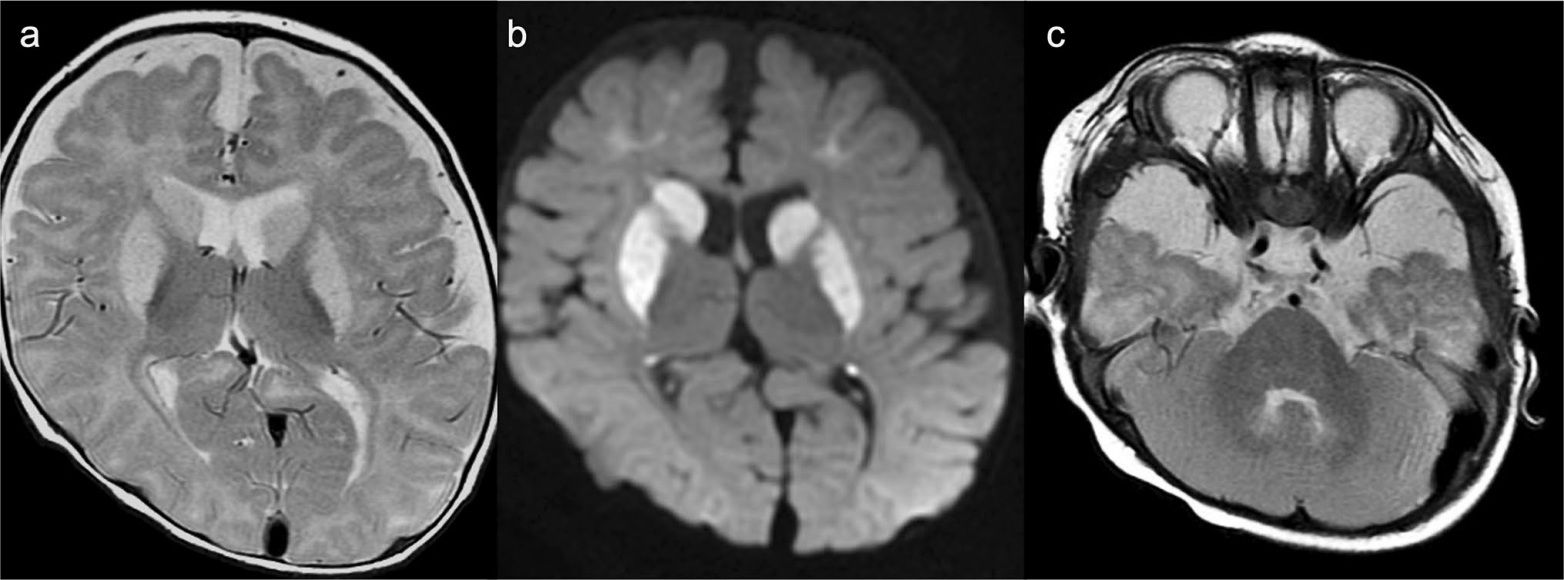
Glutaric aciduria type 1 (GA1). A 6-month-old, male patient with eye deviation, cyanosis, and myoclonus. a, b T2-weighted images (a) and DWI (b) demonstrated increased high signal and restricted diffusion of bilateral caudate nuclei and putamina. c T2-weighted images demonstrated enlargement of the Sylvian fissures caused by hypoplasia of the temporal opercula
